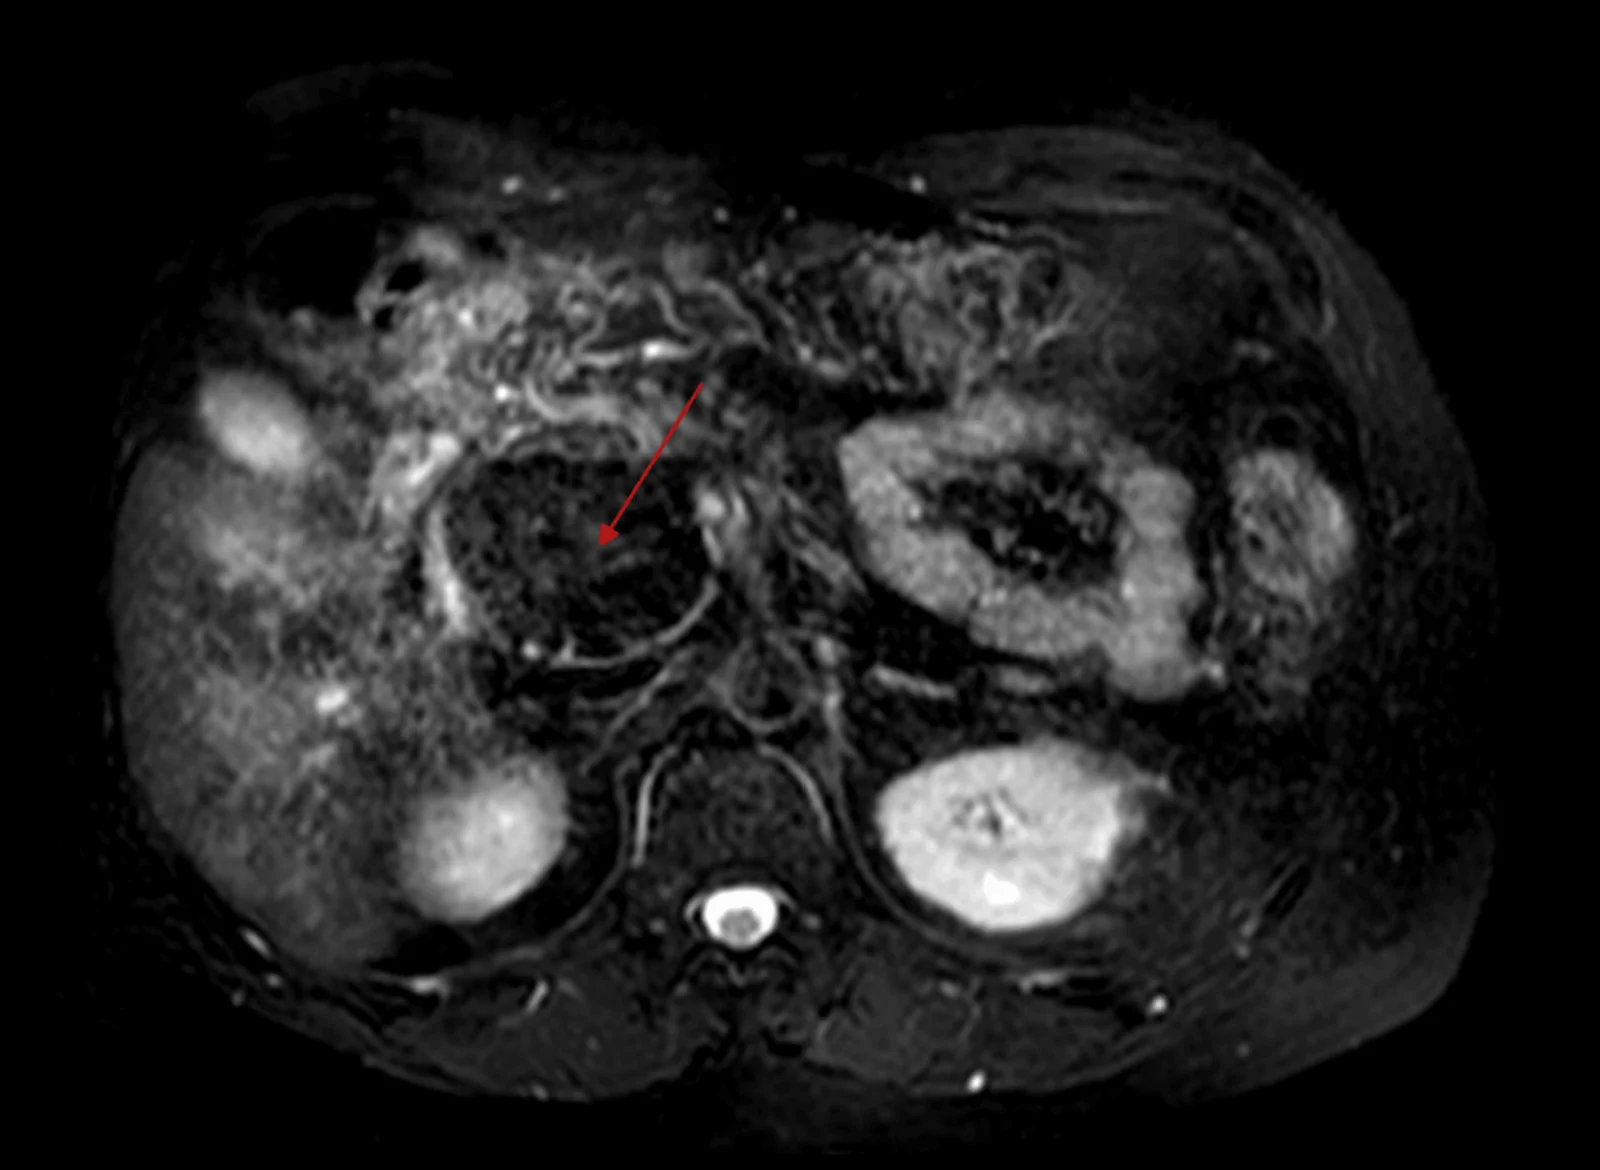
Contrast-enhanced abdominal magnetic resonance imaging, T2-weighted sequence with fat saturation (axial plane) The lesion shows homogeneous and complete loss of signal on fat-suppressed sequences (red arrow).
